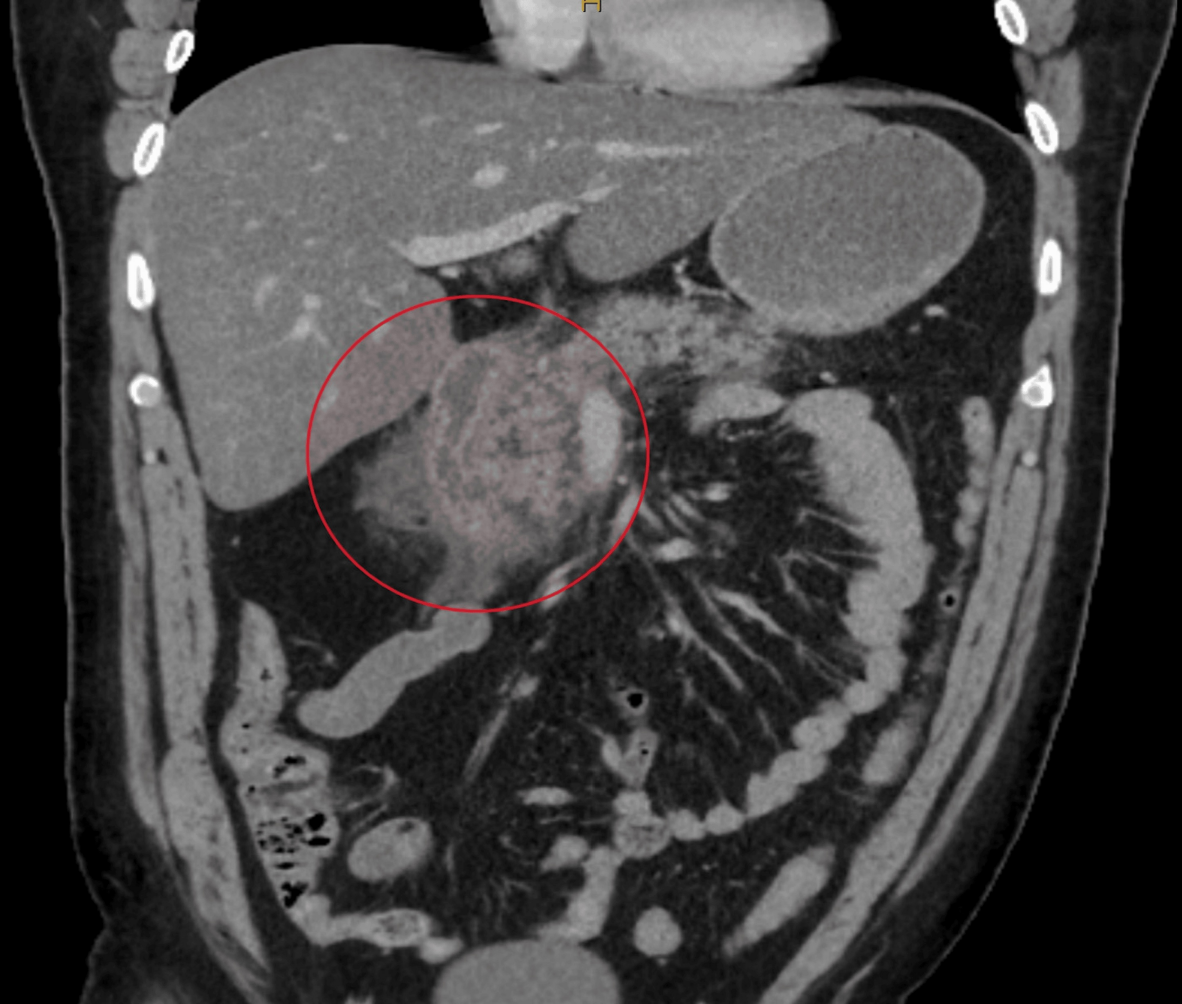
Coronal CT of the Abdomen and Pelvis Without Contrast Showing Peripancreatic Stranding, Contiguous Inflammation About the Descending Duodenum, Fluid in the Mesentery, and Hepatic Steatosis
